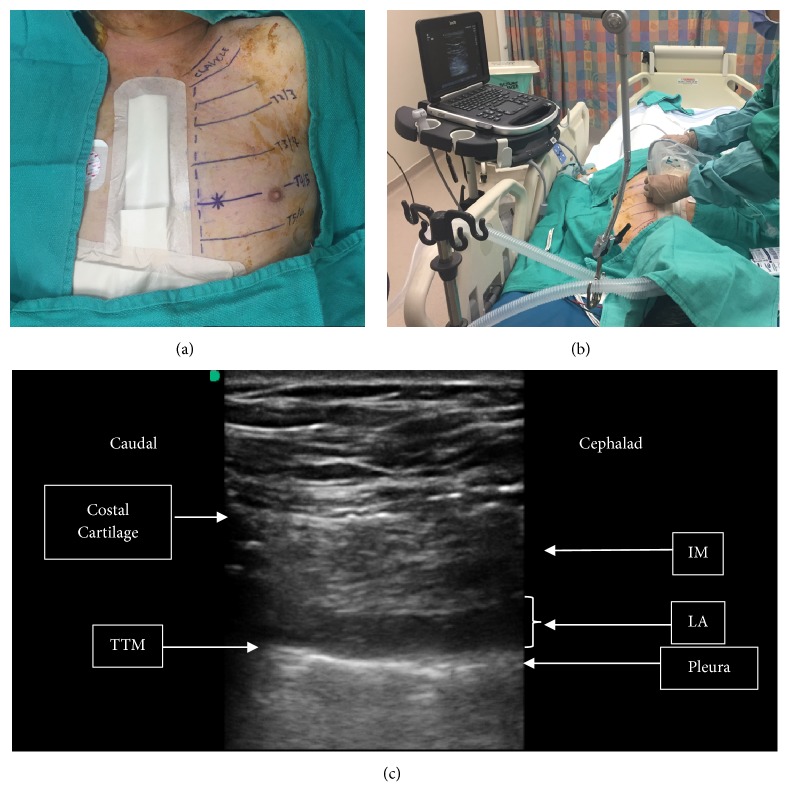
Patient positioning and ultrasound images in the TTP block. (a) Anterior chest wall of patient after cardiac surgery. The thoracic intercostal spaces are marked. The injection site is indicated by an asterisk. (b) The operator stands beside the patient with ultrasound machine on opposite side. The ultrasound probe is placed longitudinally to the sternum. (c) Ultrasound image after TTP block. The local anesthetic spreads uniformly between the IM and TTM. The pleura is displaced downwards. IM, internal intercostal muscle; TTM, transversus thoracis muscle; LA, local anesthetics.
